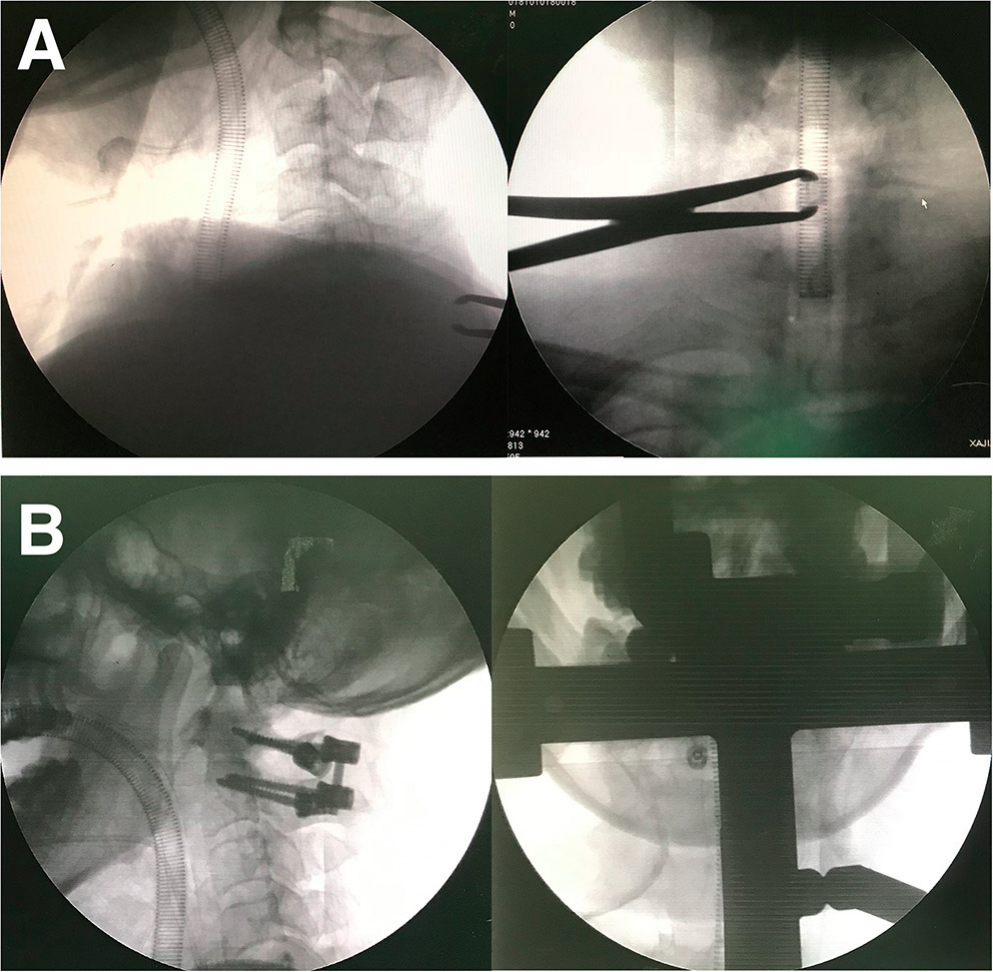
Anterior-posterior intro-operation radiography is available in BSPST position benefit from X-ray penetrable characteristics of plaster (A,B).
